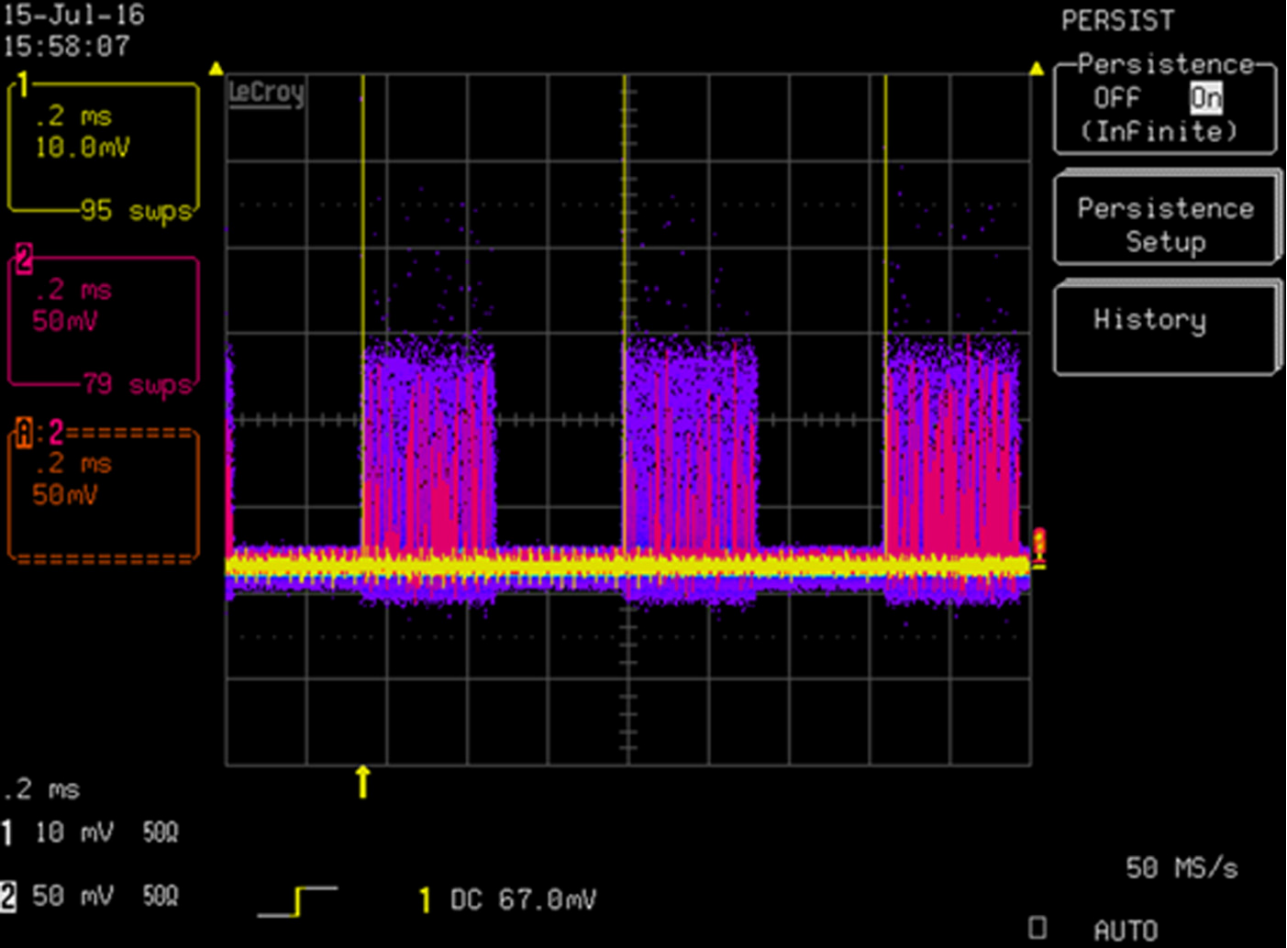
Oscilloscope view showing the X-ray period (purple) and the synchronized laser pulses (yellow) during the pump probe stage of the experiment.
